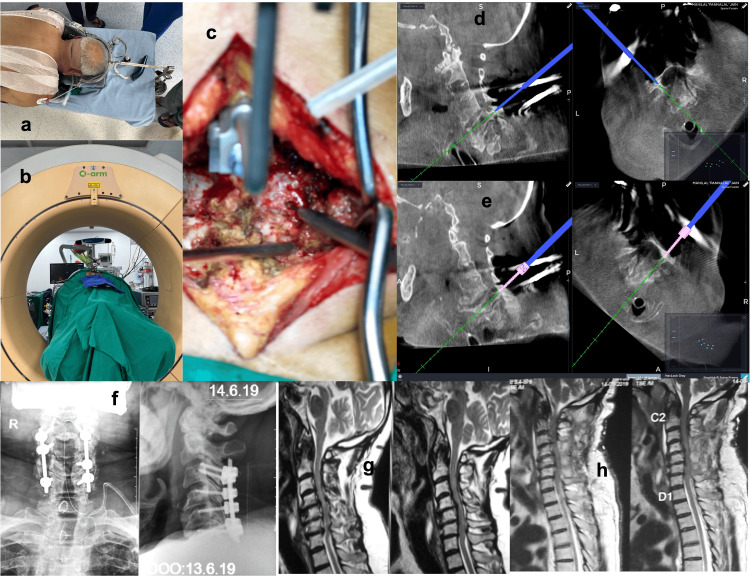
Intraoperative pictures of a male patient with cervical myelopathy Intraoperative pictures of a male patient with cervical myelopathy shows a) positioning and fixation of cervical spine with cervical tongs, b) Intraoperative CT cuts using O-arm and navigation, c) our CPEP entry point at cervical pedicle using matchhead burr, d) intraoperative cervical pedicle entry with cervical probe under navigation, e) insertion of pedicle screw with trajectory under navigation guidance, f) postoperative x-ray of cervical spine AP and Lateral view, g) preoperative T2 sagittal view of MRI comparing with h) postoperative T2 sagittal view of MRI showing improvement in kyphosis and spinal canal compression.
